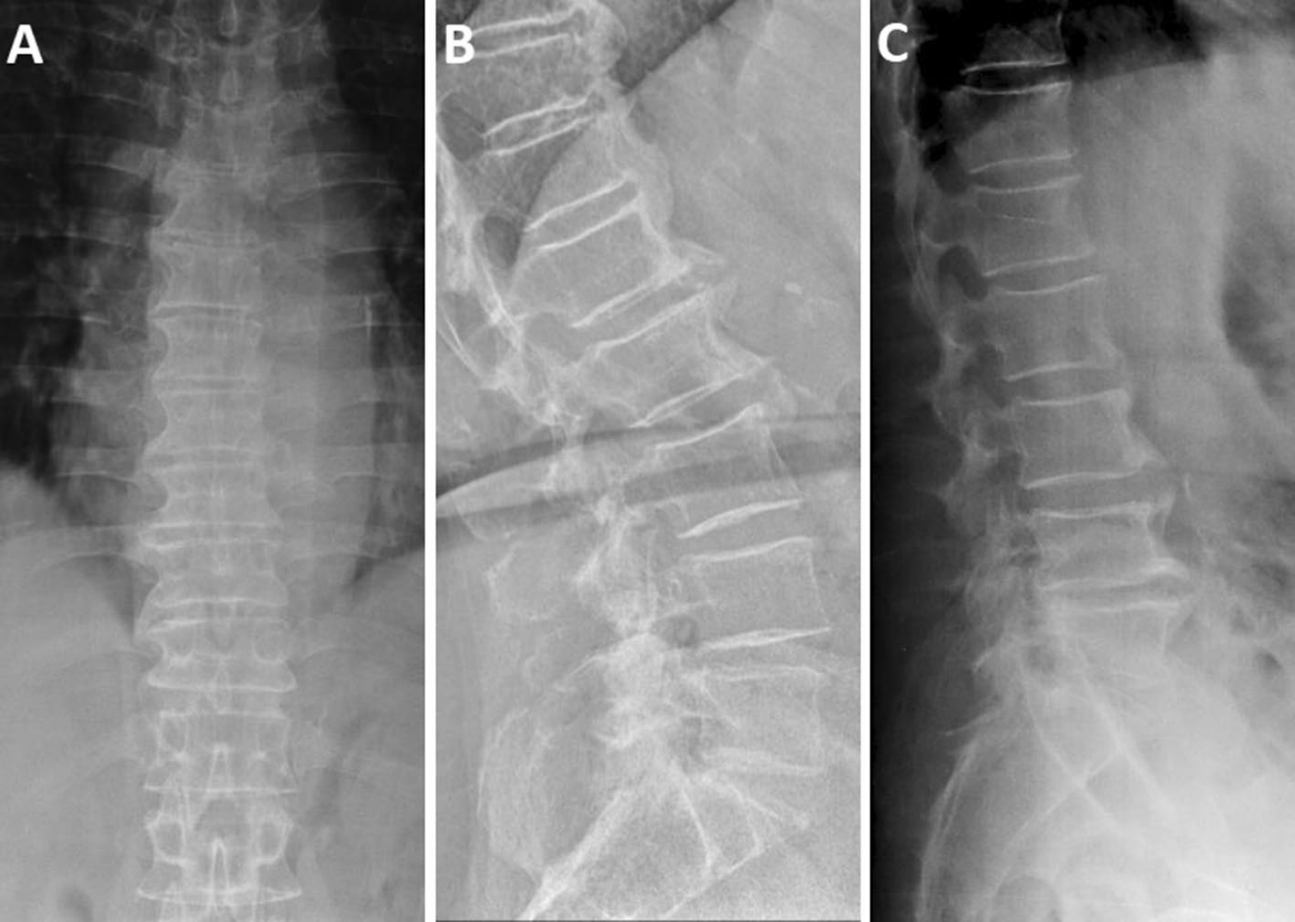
Thoracic, thoracolumbar and lumbar DISH. a Anterior–posterior radiograph showing right-sided flowing ossification of the thoracic spine; b lateral radiograph showing bone bridges and thick flowing ossification of the anterior lateral ligament of the thoracolumbar portion; c lateral radiograph showing large non-marginal ossification involving the lumbar spine segments with calcification of the anterior longitudinal ligament
